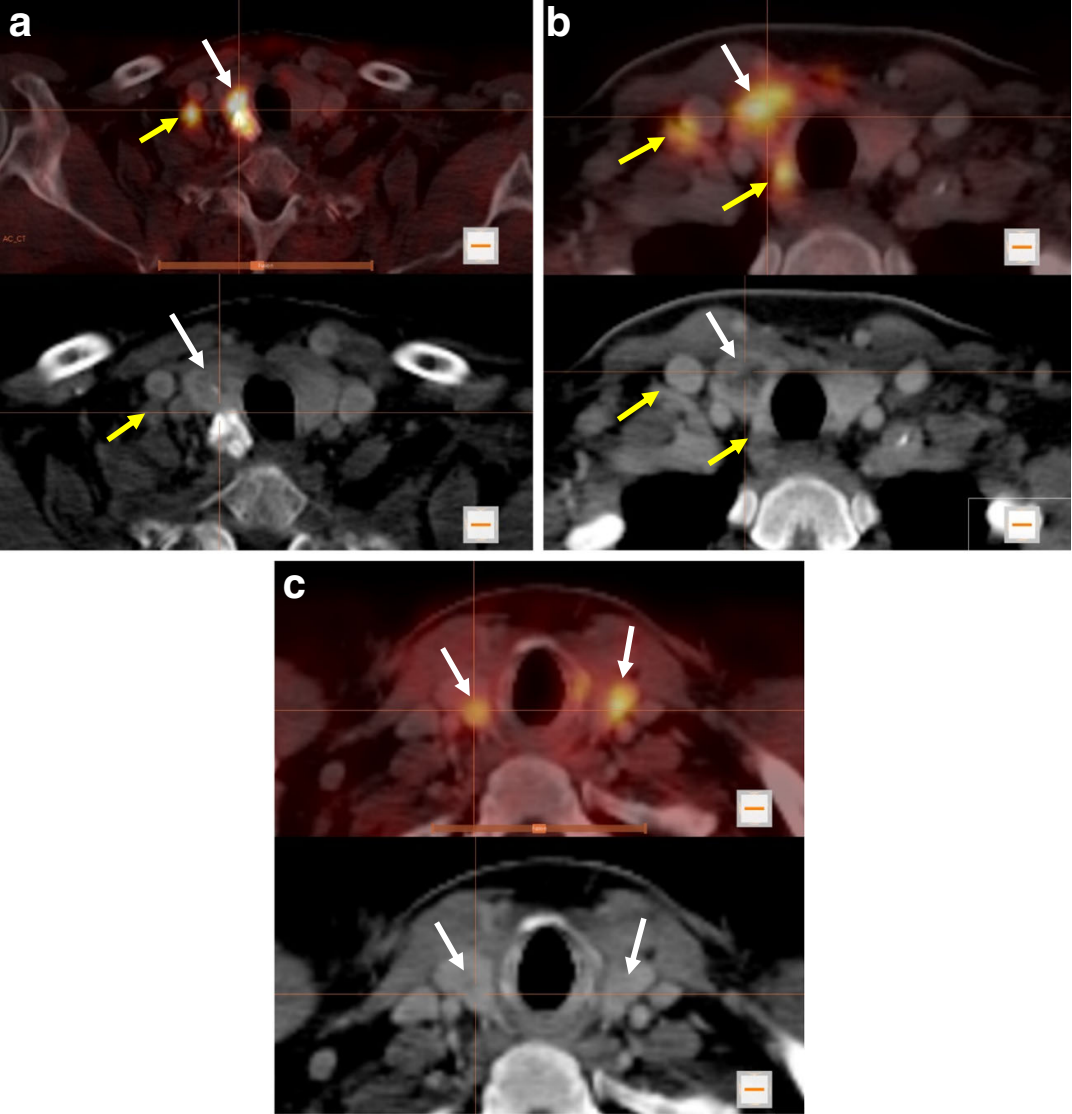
Initial [^18^F]DOPA PET/CT in patients with suspicious thyroid nodules prior to total thyroidectomy and lymph nodes dissection. a Increased DOPA uptake in the primary tumour in the right thyroid lobe (*white arrows*) and in a right lateral neck lymph node at level IV (*yellow arrows*) in a 61-year-old male patient. b Increased DOPA uptake in the primary tumour in the right thyroid lobe (*white arrows*), in a lateral neck lymph node at level IV and in a central (paratracheal) lymph node at level VI (*yellow arrows*) in a 47-year-old female patient. c Increased DOPA uptake in the primary tumour in both thyroid lobes (*white arrows*) in a 48-year-old male patient
